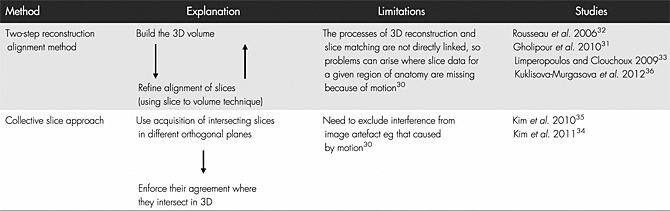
Techniques developed to overcome fetal motion and create 3D volumetric images from a stack of 2D motion frozen MRI images
